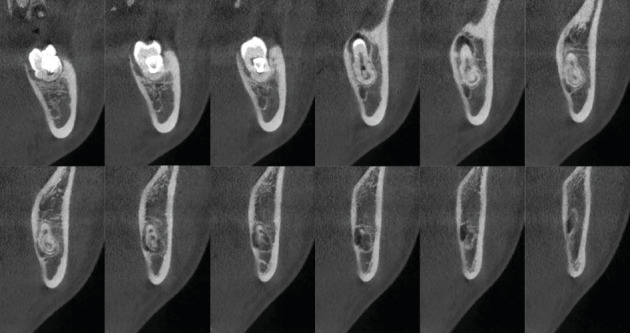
Sagittal CBCT cross-sections of the 3.8 tooth element depict the continuity relationship between the root apex and the NVB.
